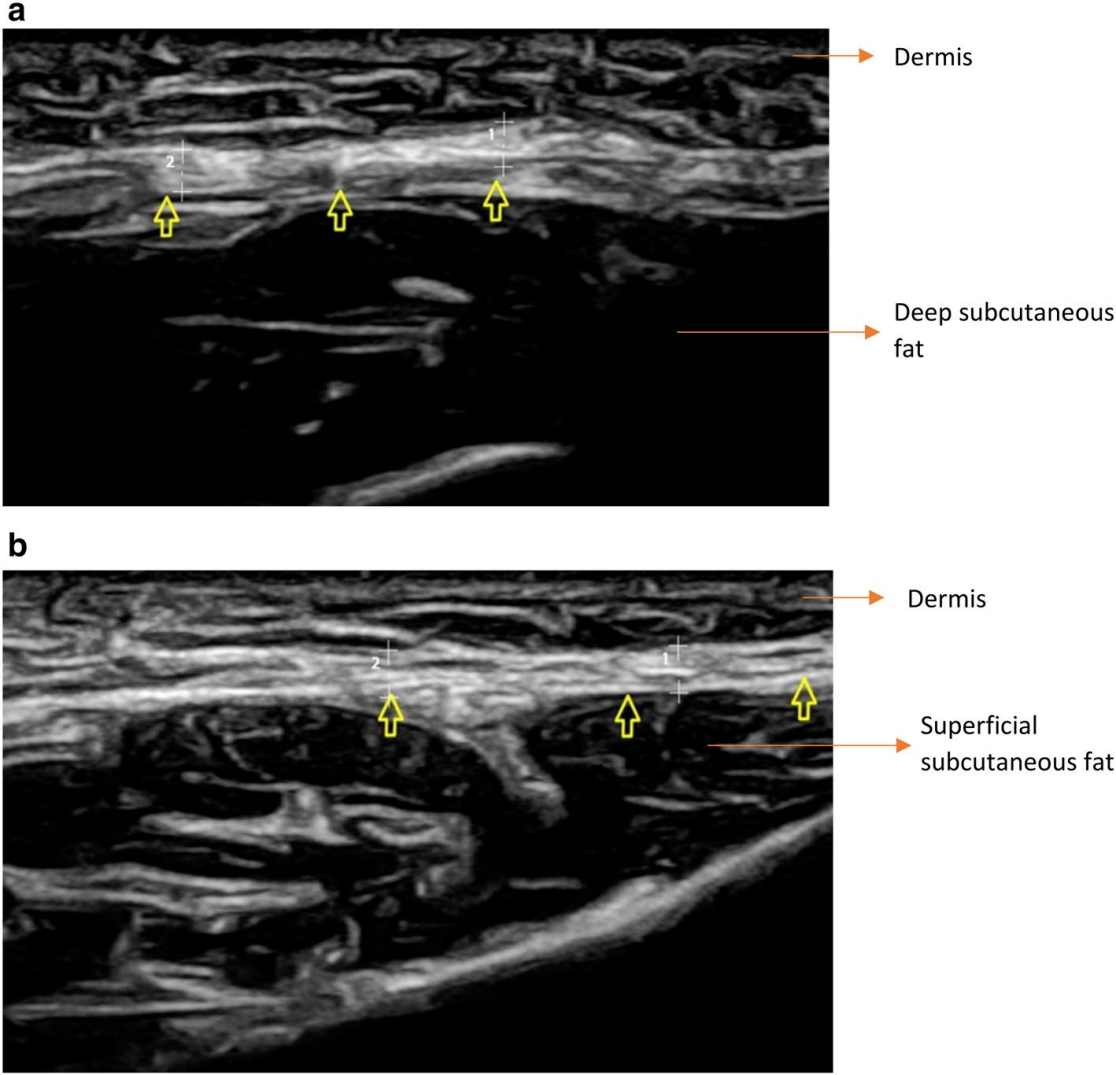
Two sonograms obtained from the midface of a case that was followed up for more than 7 years (86 months). Two different threads are shown in (a, b) indicated by yellow arrows. No changes in the placement of the threads were detected. A significant increase in thread thickness and echogenicity is clearly prominent to the eye.
